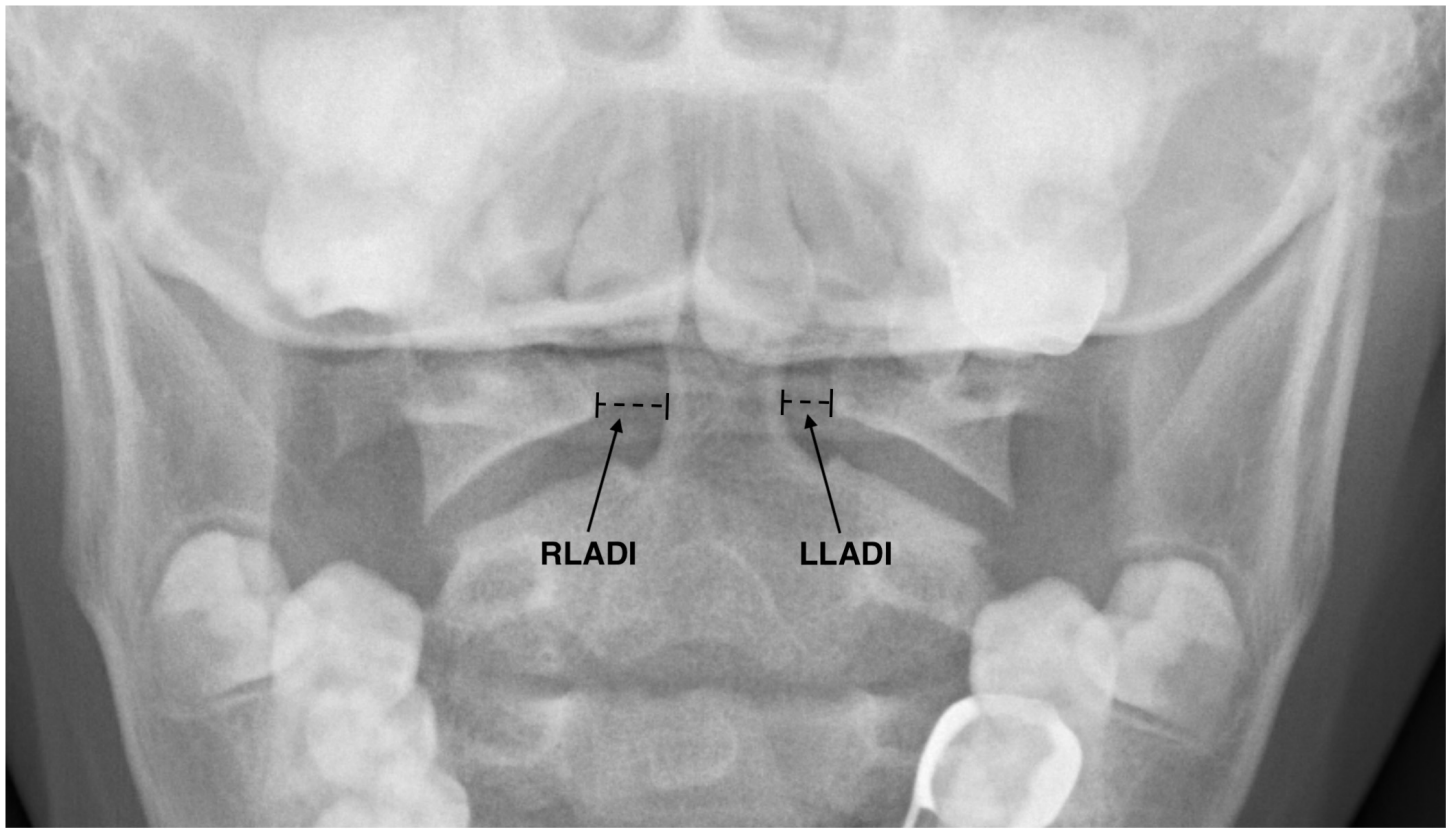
Measurement of the LADI on digital radiography. The dotted lines indicate the shortest distance from the lateral margin of the odontoid process (axis) to the medial margin of the lateral mass (atlas).
